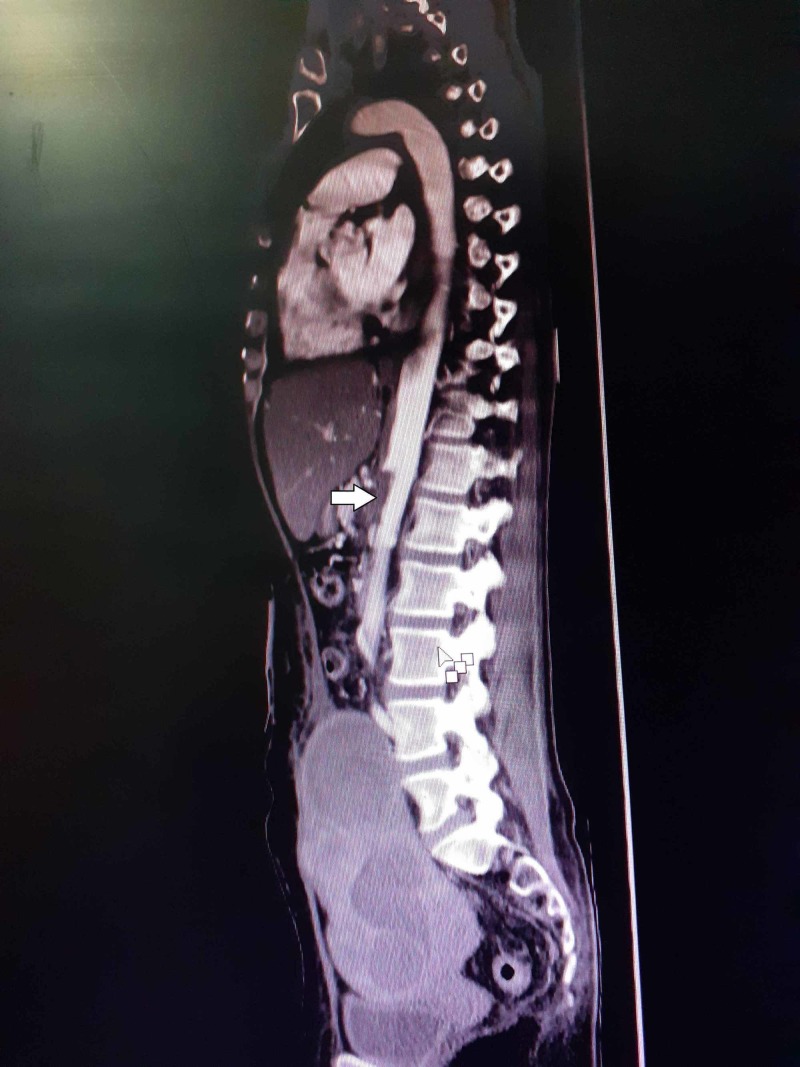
CTA showed non-dissolution of aortic thrombus at three months follow-up CTA, computed tomography angiography
